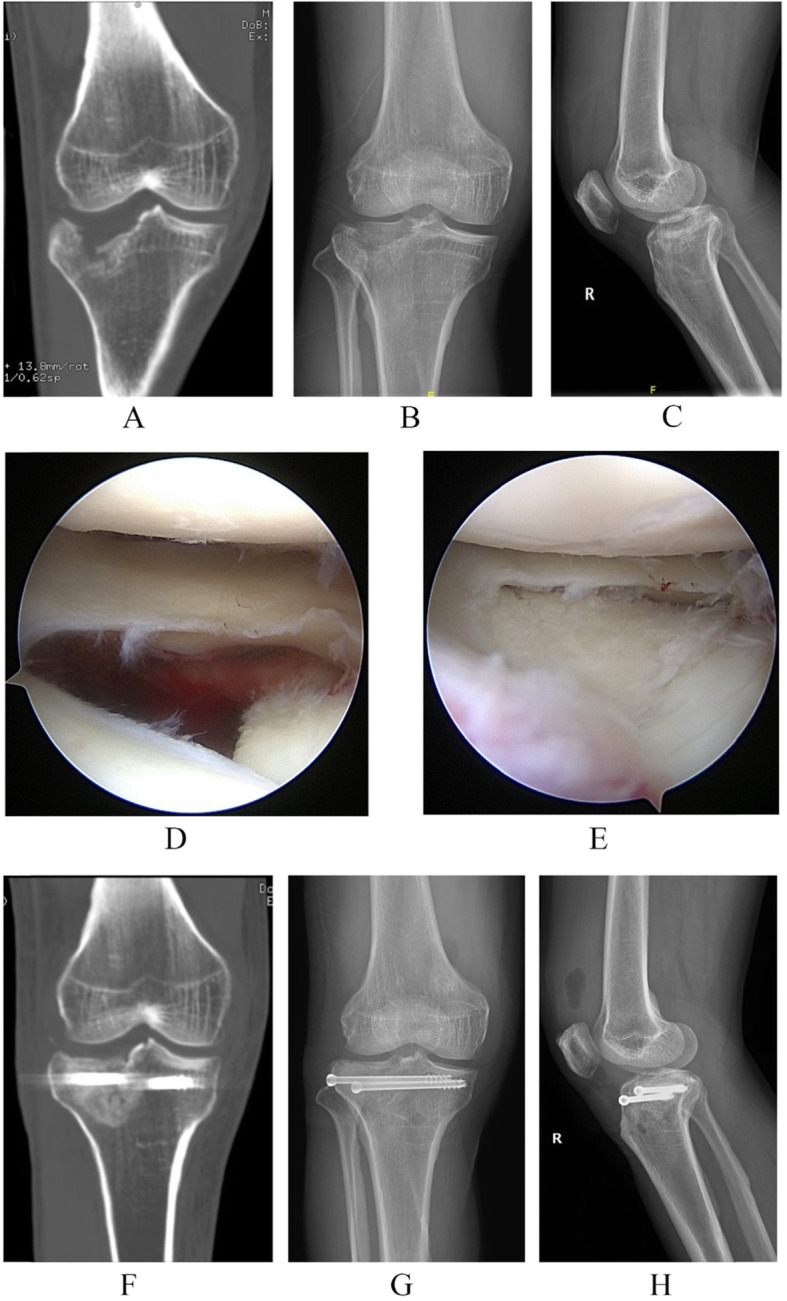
A 44-year-old male patient who was diagnosed with the lateral inclination type of posterolateral tibial plateau fracture with LI patterns. a The coronal image of three-dimensional CT scans shows the lateral inclination of the tibial fracture fragment. b The anteroposterior (AP) plain film of the injured knee. c The lateral plain film of the injured knee. d The tibial plateau fracture visualized with the assistance of arthroscopy. e After restoration of the fracture fragments with the surveillance of arthroscopy, the step-off was eliminated. f The coronal image of three-dimensional CT scans shows the restoration and the fixation. g The post-operation AP film of; H, the post-operation lateral plain film
